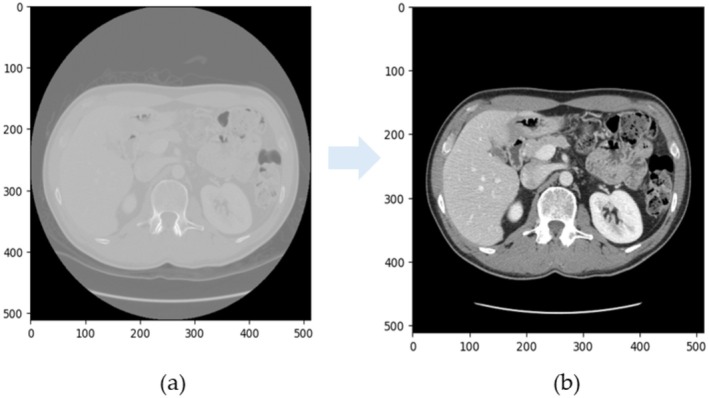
Example of a CT slice before (a) and after (b) contrast enhancement. Firstly, we convert raw pixel data values to Hounsfield Units; then we have adjusted the pixel values for visualization purposes.
